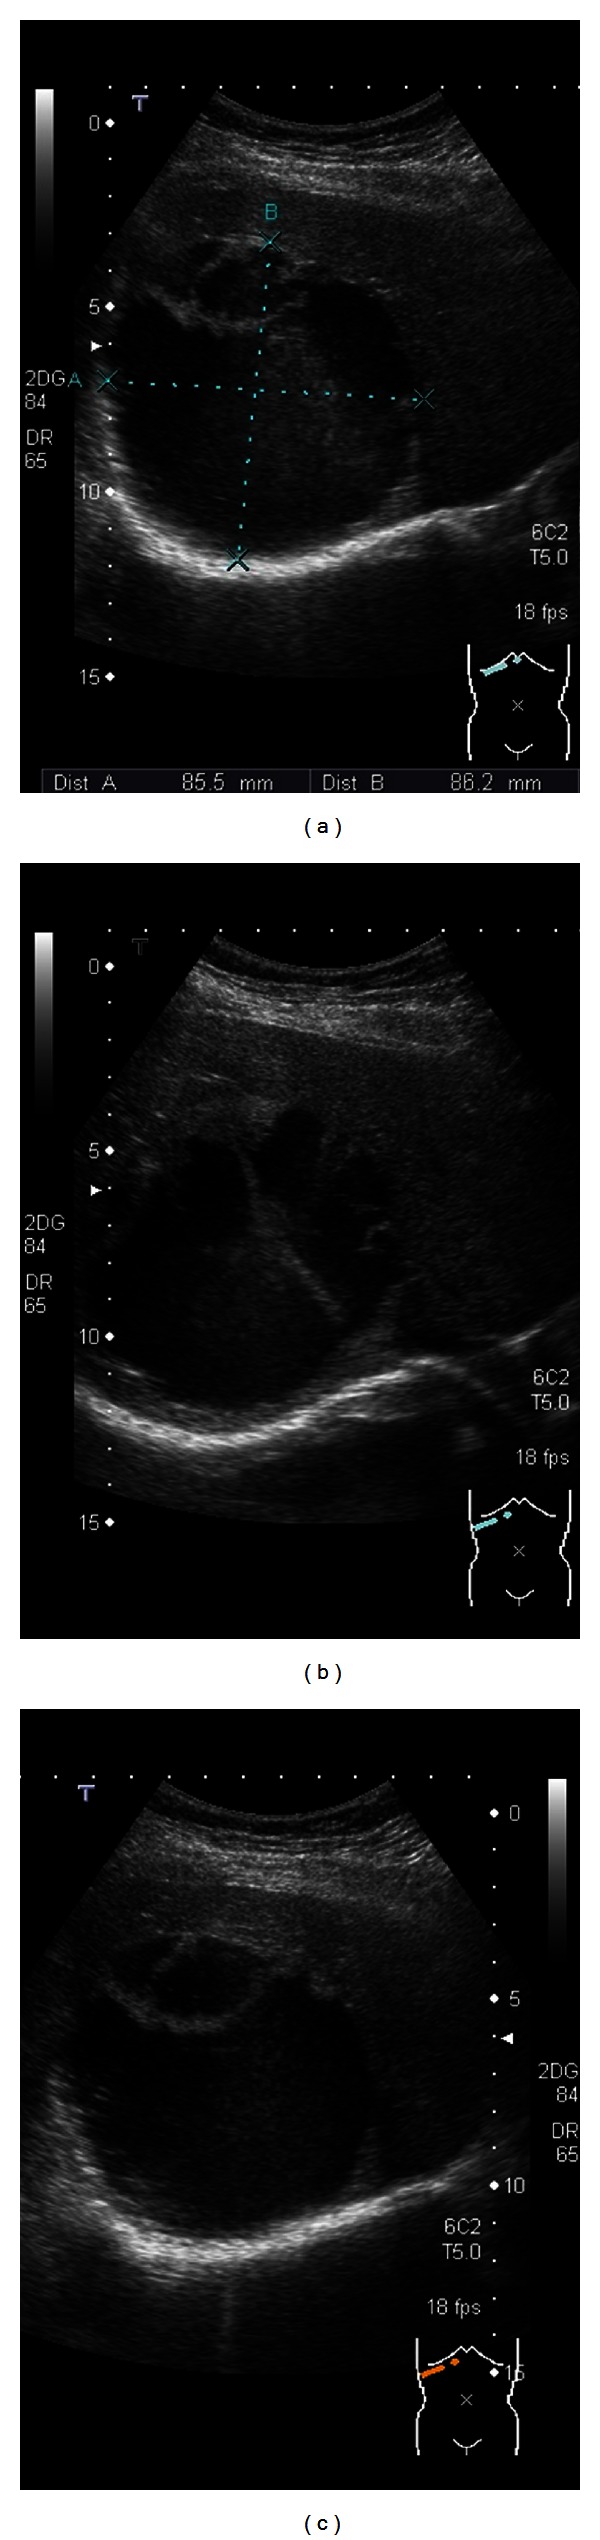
Abdominal ultrasonography performed in January 2012 reveals multiple cysts in both hepatic lobes. The cystic lesion occupying the entire right hepatic lobe shows irregular septal structures and nodules.
